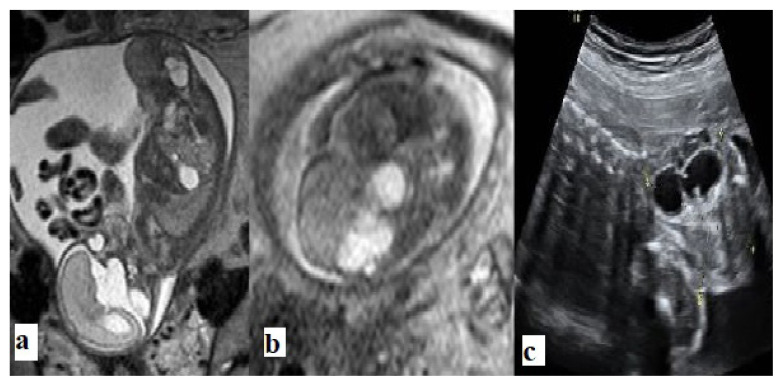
Type III sacrococcygeal teratoma. a. Sagittal, and b. Axial T2-weighted, c. Ultrasound images show large mixed signal intensity tumor extending into abdomen.
